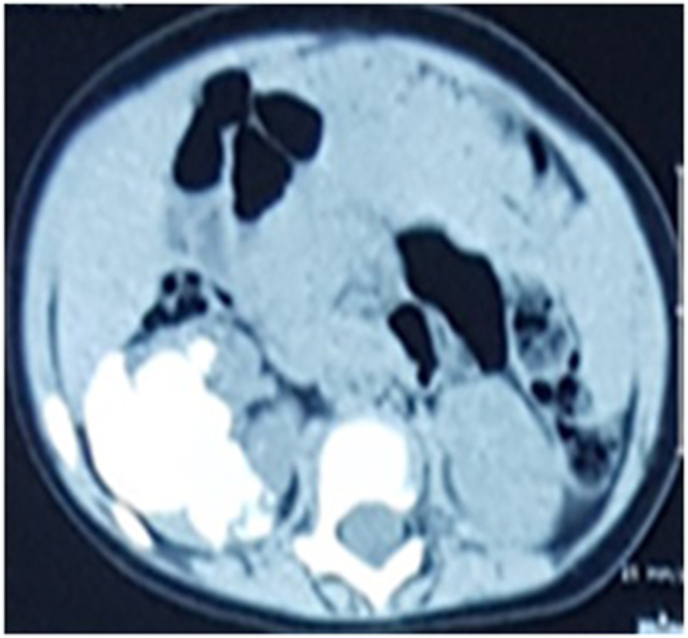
Axial nonenhanced CT image shows a calcified renal mass located in the upper pole and central part of the right kidney with no tissue portion visible.
